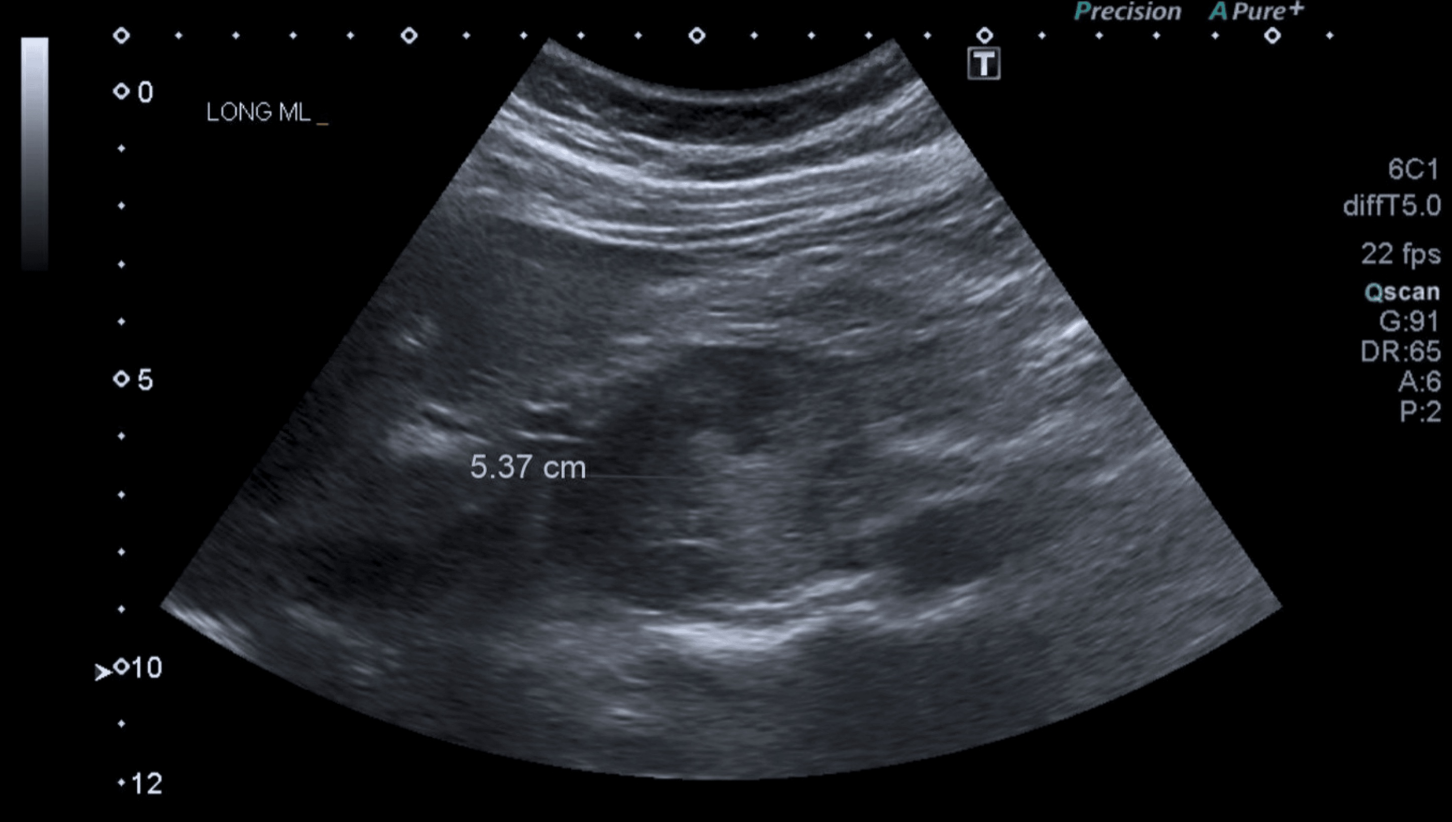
Abdominal ultrasound showing a pancreatic head mass
